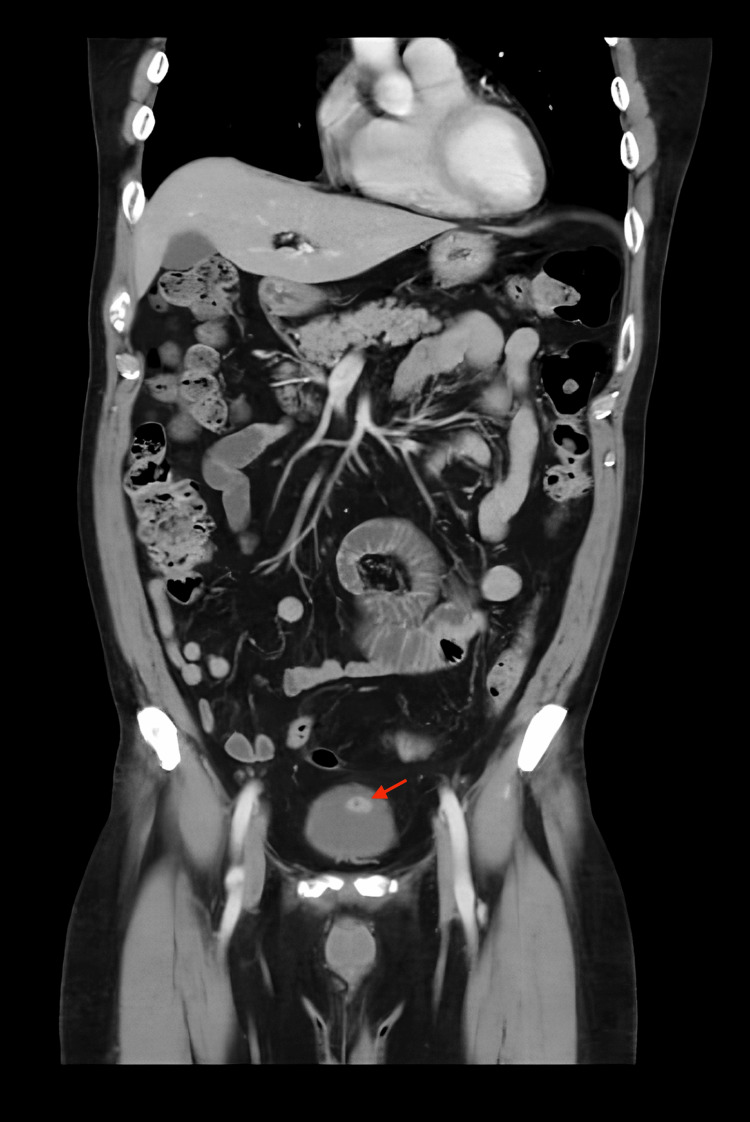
Contrast coronal abdomenipelvic CT scan demonstrating an enhancing mural nodule on the anterior bladder wall suggestive of malignancy. The red arrow in the image points to an enhancing mural nodule on the anterior bladder wall measuring 1.0 × 1.7 × 1.0 cm, with prominent feeding vessels arising from the left inferior vesical artery.
